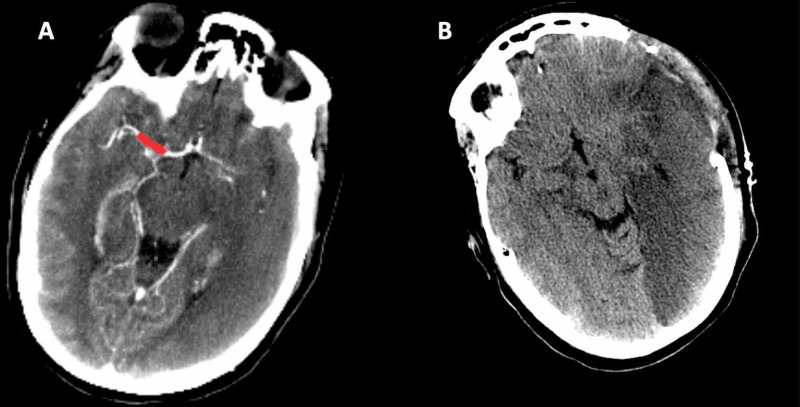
(A) CTA reveals acute thrombosis of left M1 segment with large left MCA malignant infarct. (B) Post-hemicraniectomy CT showing malignant left MCA infarct with craniectomy noted. CTA: Computed tomography angiography CT: Computed tomography MCA: Middle cerebral artery
